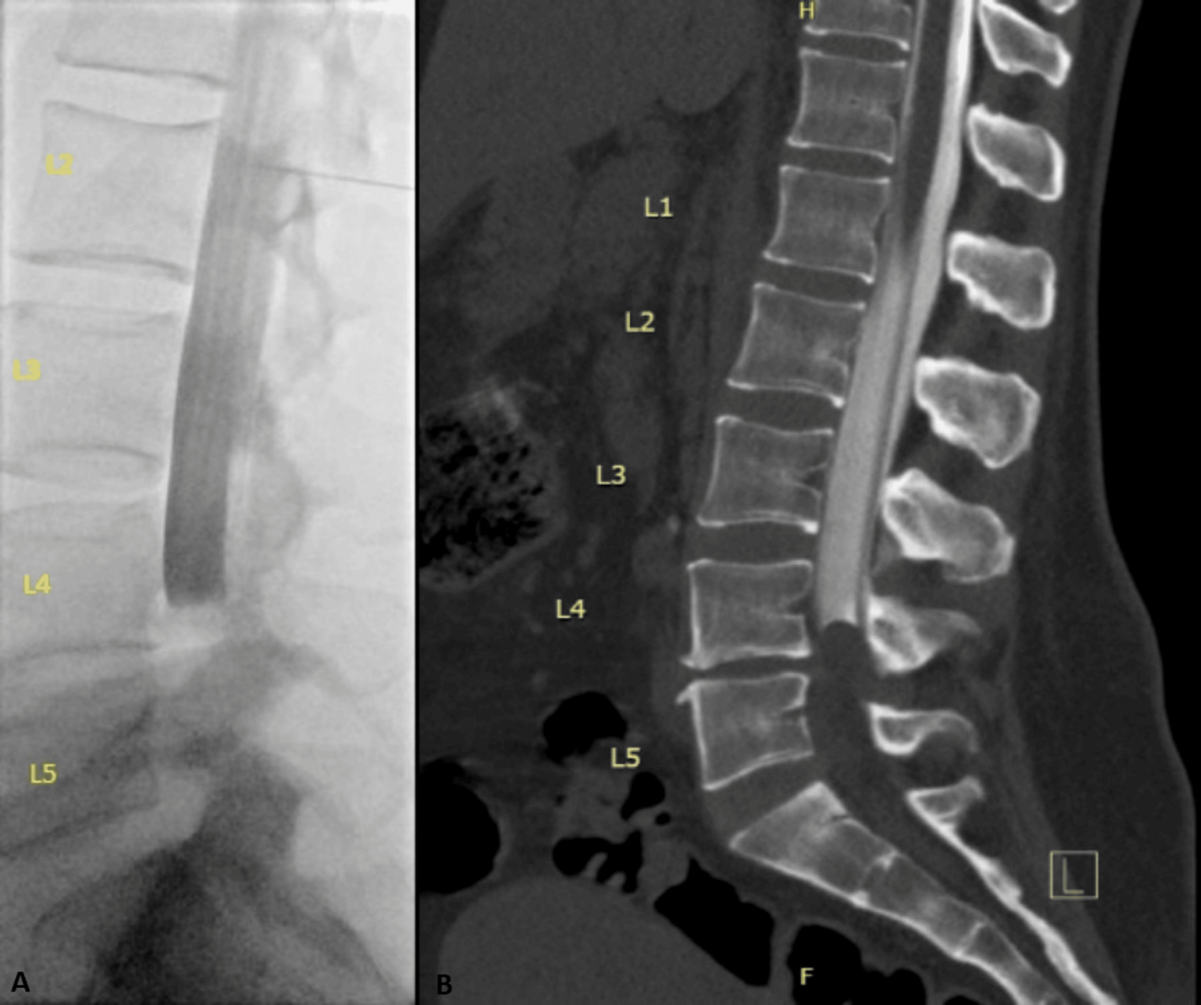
Confirmation of arachnoid cyst beginning at L4. From left to right, these images confirm the presence of an arachnoid cyst. Image A is an X-ray myelogram showing intrathecal injection of contrast material for a CT lumbar myelogram. Image B is a CT lumbar myelogram demonstrating complete attenuation of contrast enhancement below the mid-4th lumbar vertebra.
